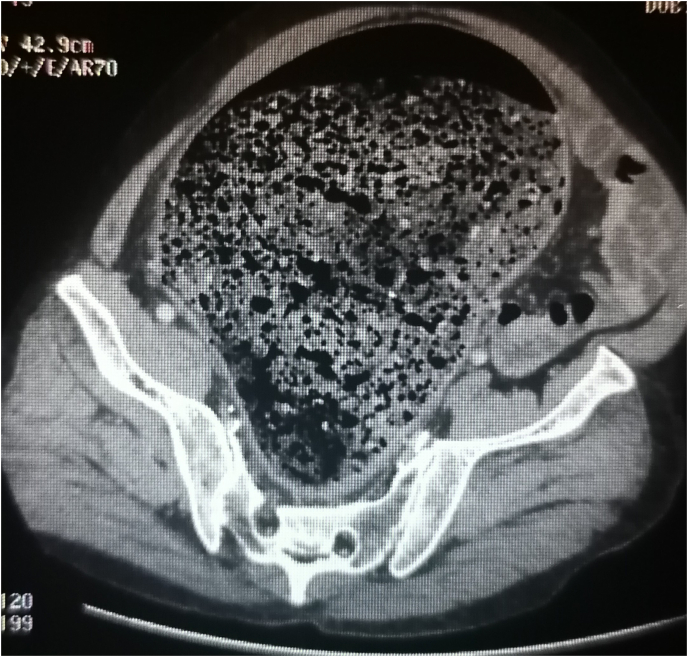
CT scan on admission showing a very dilated sigmoid colon filled with faeces.
